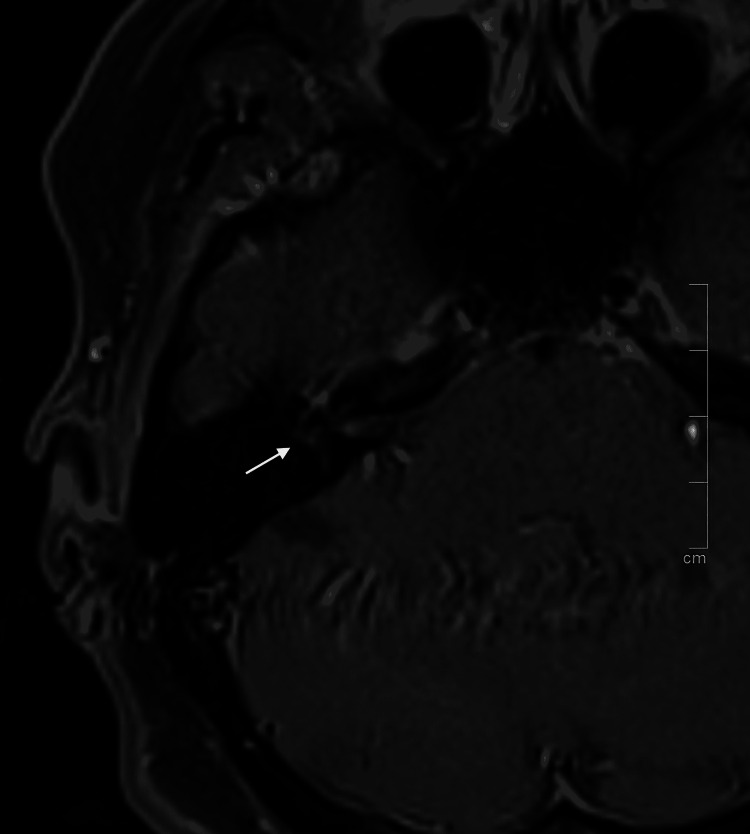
Head magnetic resonance imaging. T1 hyperintensity after gadolinium enhancement of the right-sided cochlear basal turn and geniculate ganglion and enhanced signal of vestibulocochlear bundle bilaterally (white arrow).
